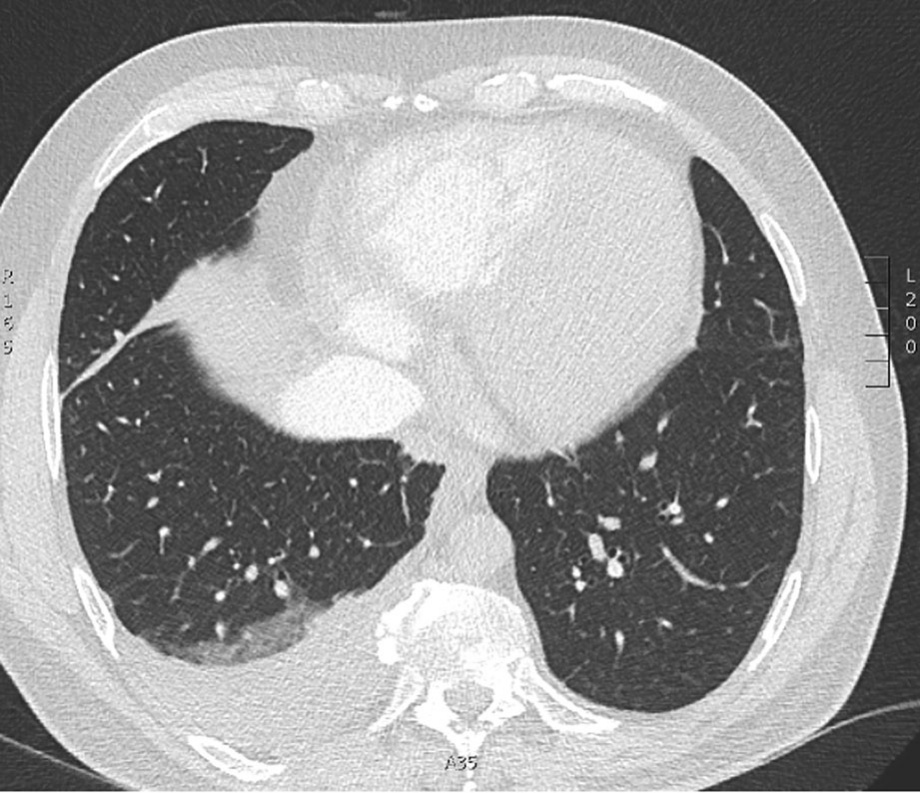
Computed tomography of the thorax. Lung parenchymal nodules and nodal enlargement in keeping with sarcoidosis. Cardiomegaly and right-sided pleural effusion secondary to decompensated cardiac failure
